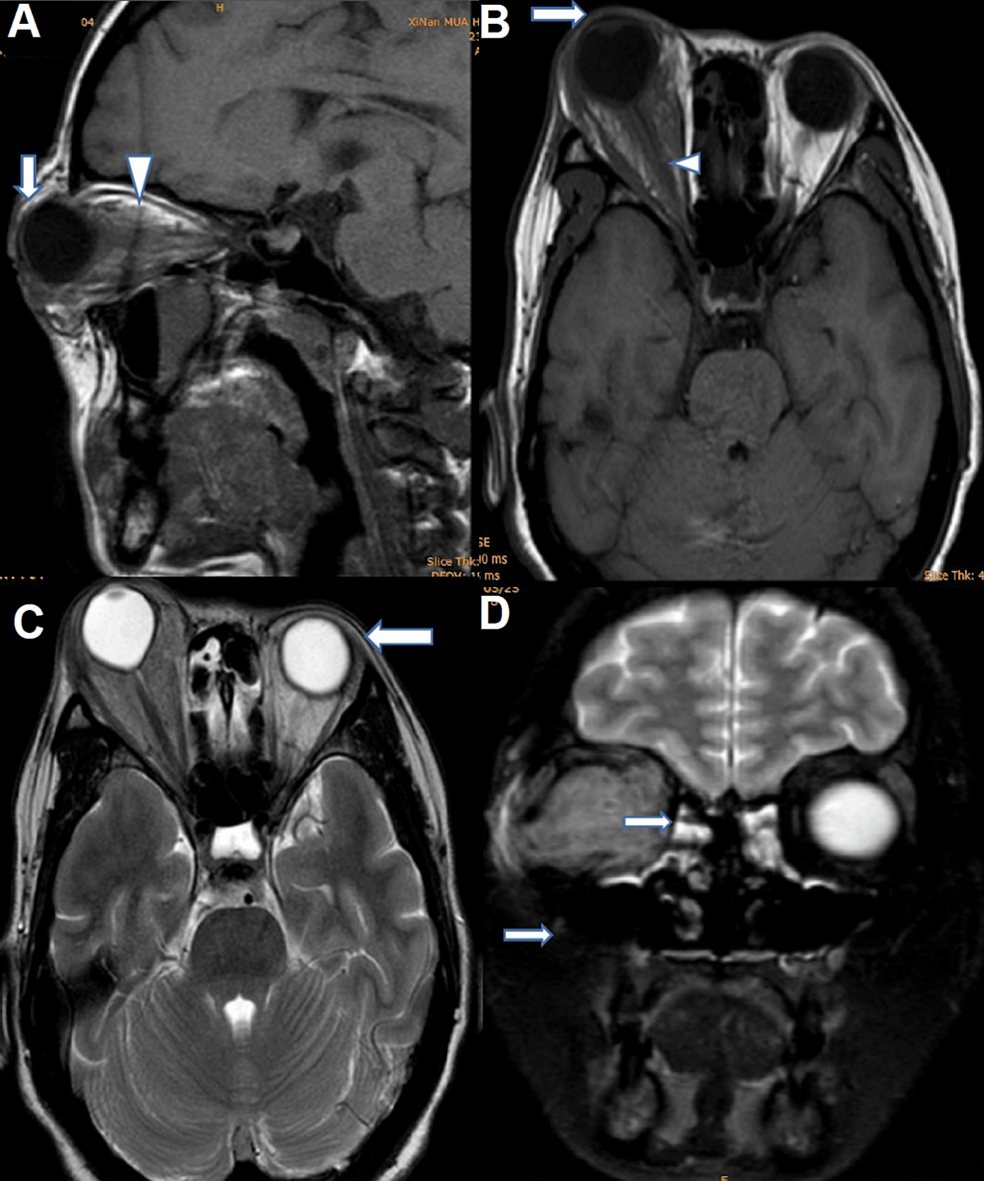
Binocular MRI sagittal, coronal, and axial planes A) Right eyeball prominence with abnormal shape (arrow) due to enlarged anteroposterior diameter, extensively swollen peri and retro-orbital adipose tissue (arrowhead); B) Intact eye ring with normal intra spherical lens, vitreous and anterior chamber morphology (arrow), without thickening in the muscles and optic nerve (arrowhead); C) Left eye shows no abnormality; D) condensed bilateral maxillary, and ethmoid sinuses and effusion are seen on right maxillary and sphenoidal sinuses.
